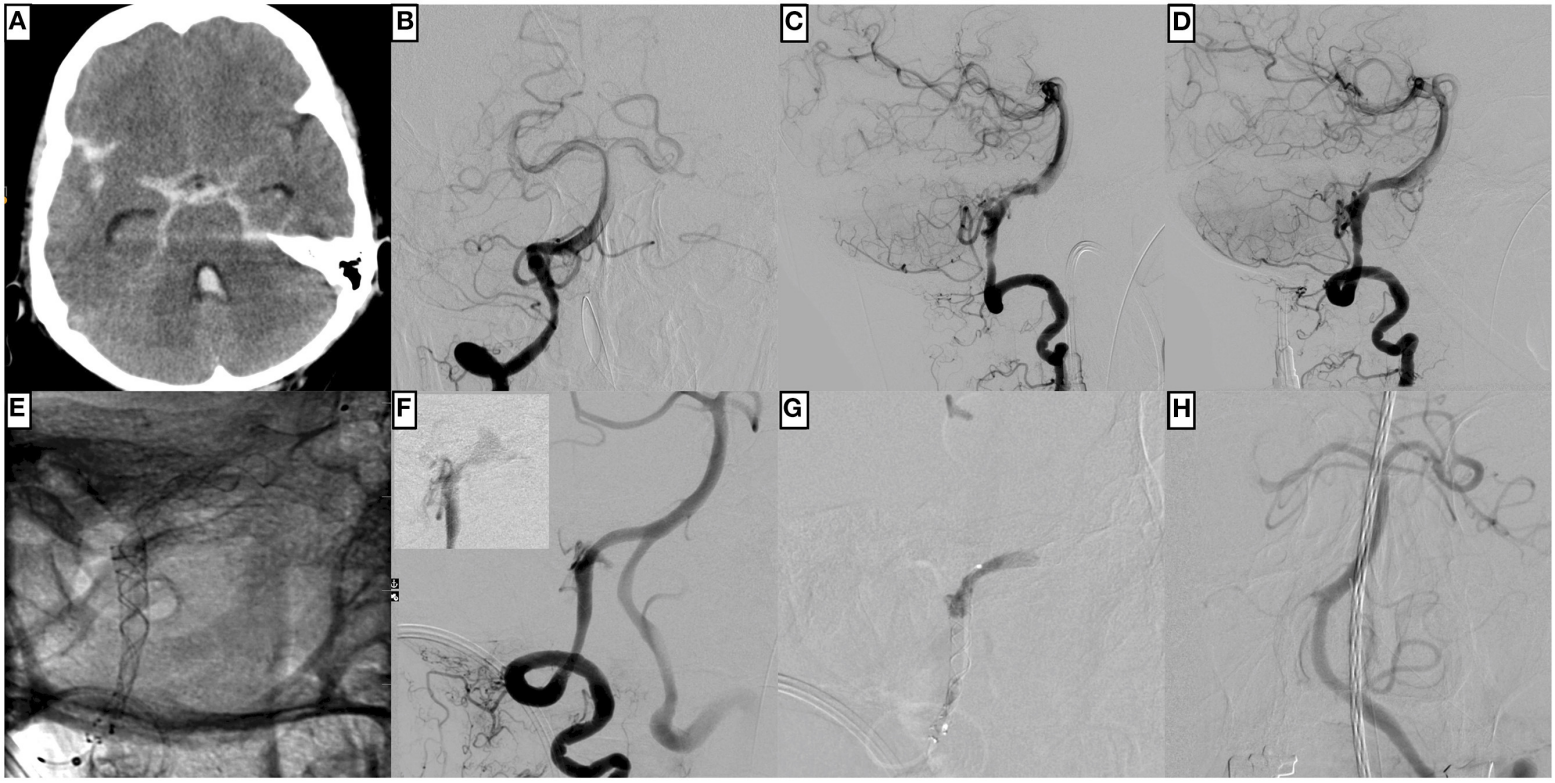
An example of complicated p64 implantation for treating a ruptured, dissecting aneurysm of the right dominant intradural vertebral artery in a 66-year-old male patient. (A) Non-enhanced cranial computed tomography shows Fisher grade 4 subarachnoid hemorrhage. (B) The injection of the right vertebral artery in the posterior–anterior and (C) lateral projection demonstrates the underlying fusiform dissecting aneurysm with the PICA at its center. (D) represents the working projection for flow diverter implantation, which is subsequently evaluated in (E); two p64 are implanted in telescoping technique with sufficient overlap of both devices at the center of the dissecting aneurysm. Note the distal intraluminal position of the p64 wire and the olive at its tip. (F) shows the control injection moments after unremarkable p64 implantation, revealing significant contrast extravasation from the distal V4 segment (the smaller image in the upper left corner shows extravascular pooling of contrast agent seconds later). The procedural re-rupture after flow diverter implantation prompted embolization of the respective segment with Histoacryl, which immediately stopped the bleeding (G). A small proportion of the liquid embolic agent dislocated into the right posterior cerebral artery. The control injection *via* the left vertebral artery (H) demonstrates the basilar artery's timely perfusion and branches, except for the right posterior cerebral artery, which exhibits a slightly delayed filling.
